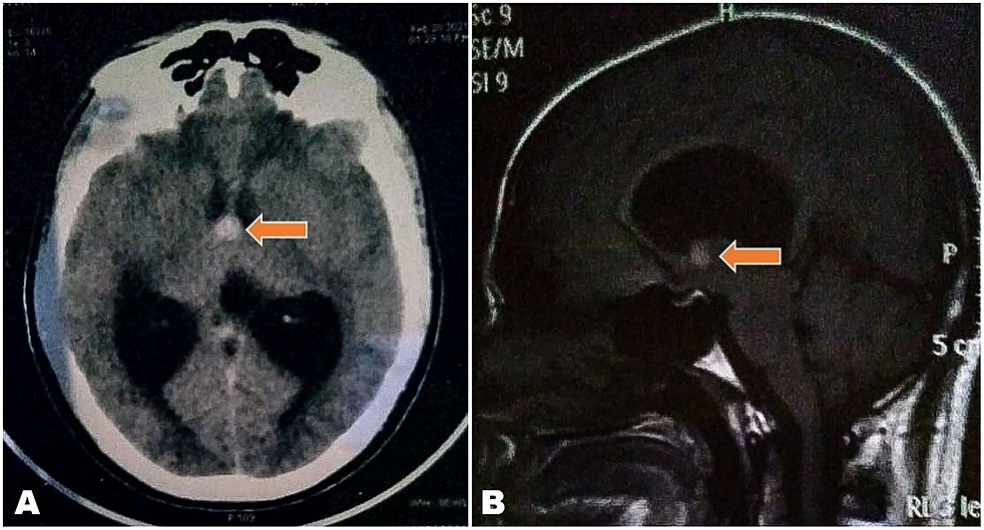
Brain CT Images A) Axial plain CT image showing dilation of bilateral lateral ventricles with a well-defined cyst in the region of the foramen of Monro with hyperdense contents within, suggestive of a colloid cyst. B) Sagittal T1-weighted non-contrast image shows a hyperintense well-defined cyst in the region of the roof of third ventricle causing obstructive hydrocephalus and effacement of the third ventricle.
